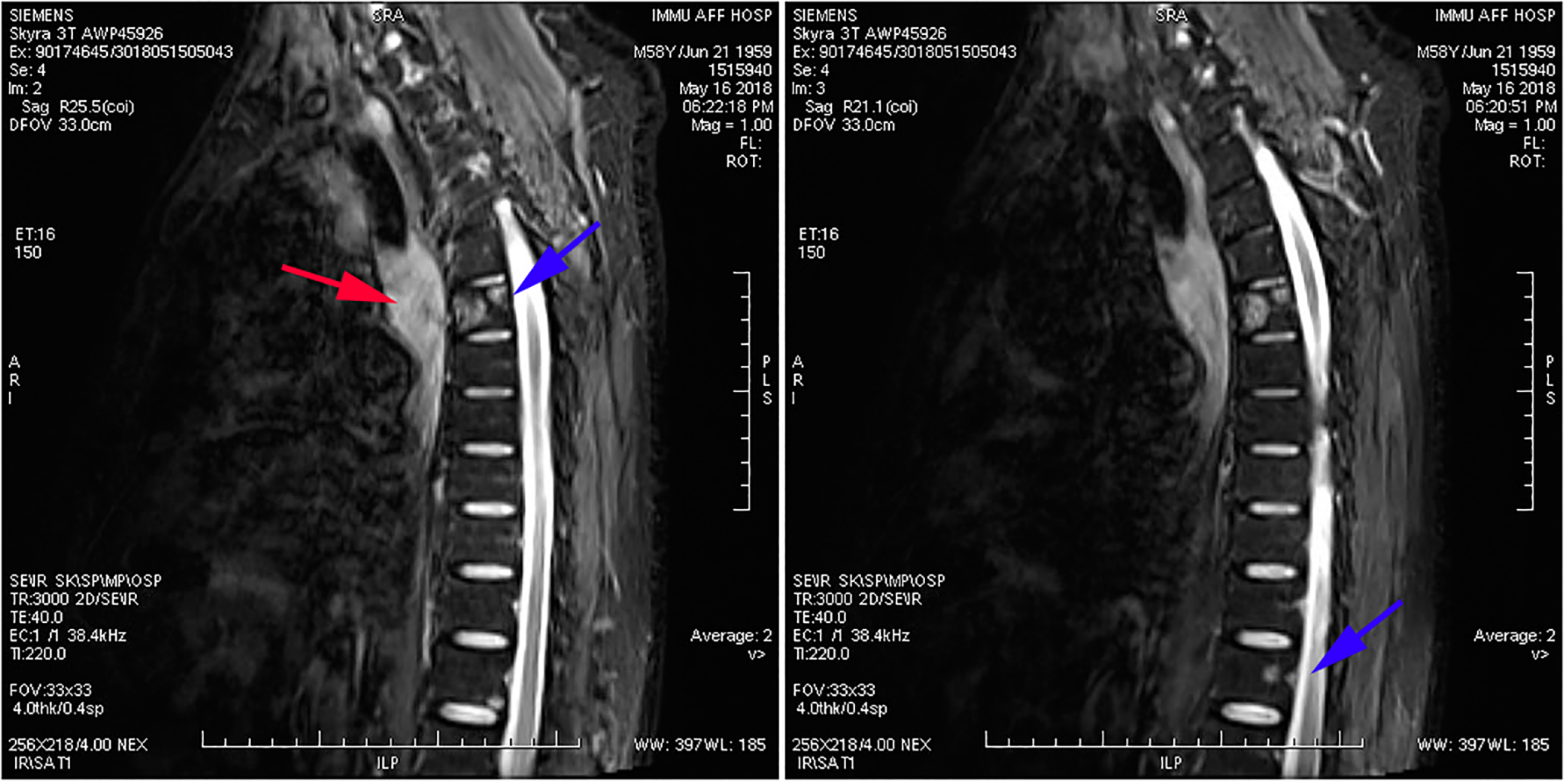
Spinal MRI demonstrated remarkable enhancement of the thorax in front of 5–6 centrum (as indicated by the red arrow), and revealed T6, and T12 destructive vertebral body lesions (indicated by blue arrows).
